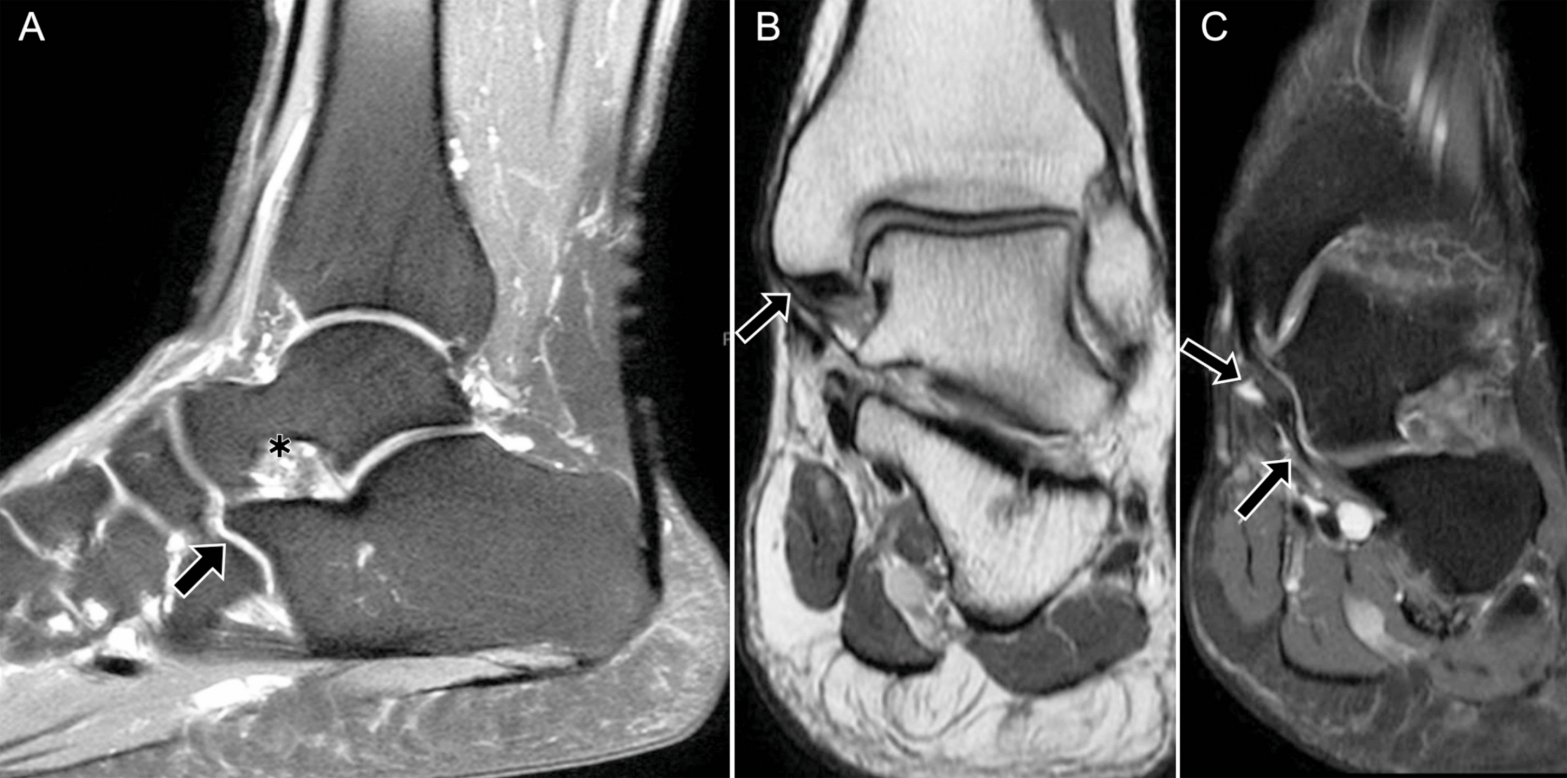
Deltoid and tibiospring ligament injuries. A 43-year-old female: (A) sagittal T2 fat-saturated MRI shows a heterogeneous sinus tarsi (*) and fluid in the calcaneocuboid joint (black arrow); (B) coronal DP MRI (double-echo proton) shows the tibiospring ligament origin (black arrow); (C) coronal DPFS MRI shows a partial superficial tibiospring tear with increased signal and laxity (arrows). DPFS: dual proton fat-saturated sequence Images belong to the authors; patient consent was obtained.
